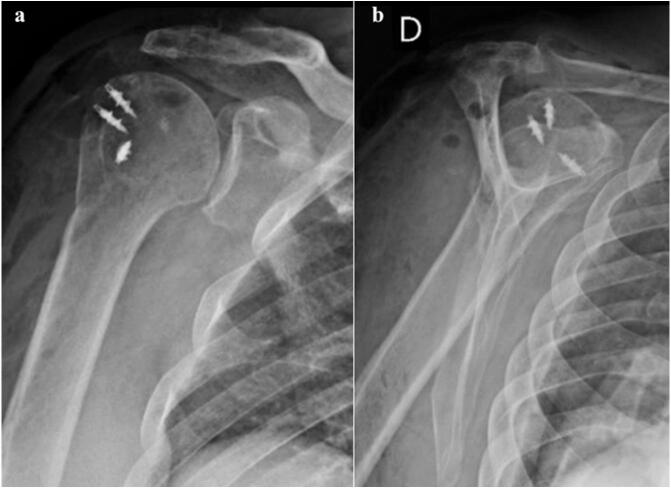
Post operative radiographic study of the right shoulder: AP view (a) and Lateral view (b): No signs of subluxation are identified.
